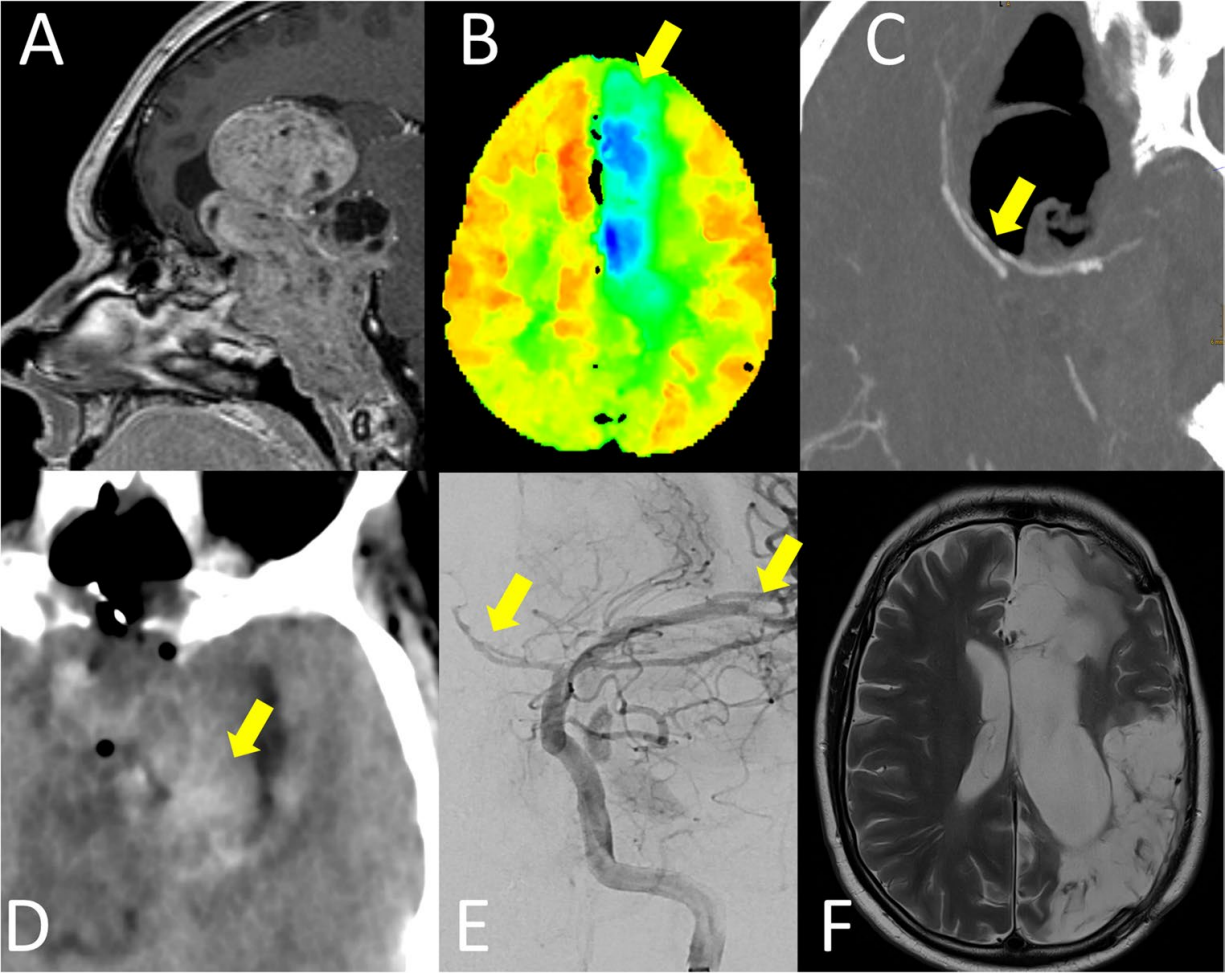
Case 3–45 year old male with giant silent corticotrope pituitary neuroendocrine tumor. A: preoperative sagittal contrast enhanced T1-weighted MR section showing extensive tumor infiltration of the anterior and middle skull base and clivus as well as a large suprasellar extension into the frontal lobe and third ventricle. B: Tmax map of perfusion CT on first postoperative day, blue areas indicating delayed perfusion, green yellow and red areas indicating timely perfusion, arrow pointing out perfusion deficit in the area supplied by the left anterior cerebral artery. C: Sagittal Reconstruction of contrast-enhanced CT angiography on postoperative day 1, arrowhead indicating focal interruption of left pericallosal artery. D: axial CT without contrast agent on postoperative day 1, arrow marking tumor bed hemorrhage predominantly on the left side. E: digital-subtraction angiography of the left internal carotid artery with arrows indicating reduced caliber of the anterior cerebral artery and interruption of the middle cerebral artery as signs of vasospasm. F: T2-weighted axial MR section demonstrating ischemic infarction of left anterior and middle cerebral artery territory
